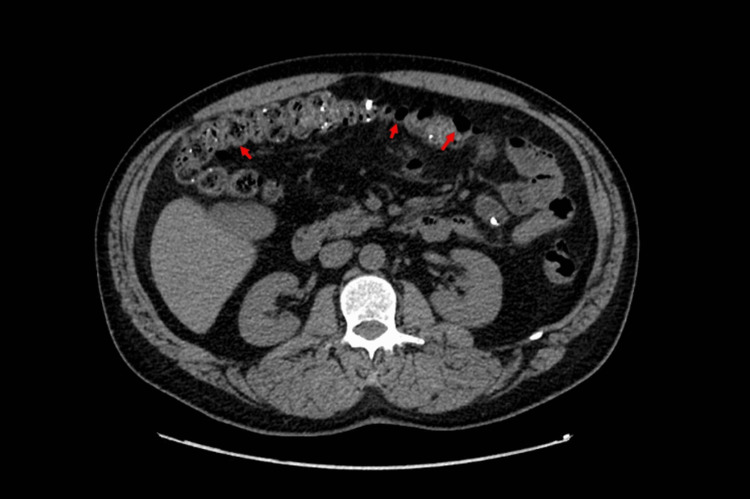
CT abdomen showing diverticulosis of the entire colon (red arrows)
